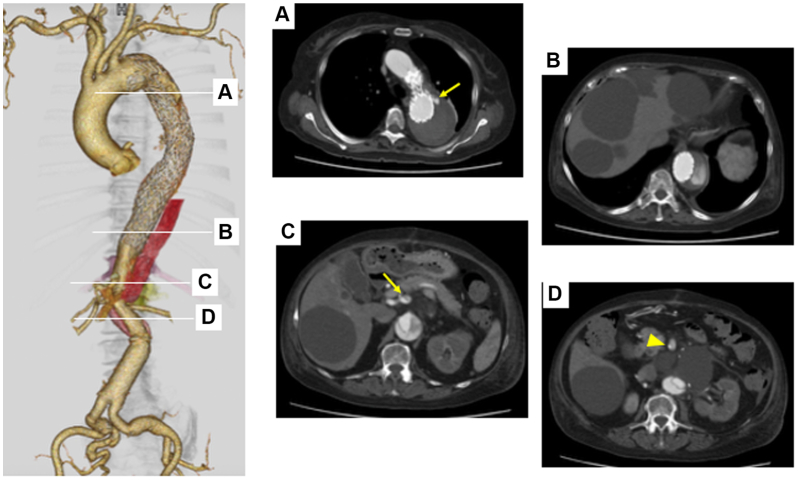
Contrast-enhanced computed tomography (CT) reveals progressive false lumen (FL) enlargement due to a type Ia endoleak after thoracic endovascular aortic repair (TEVAR) (A, *arrow*) and persistent retrograde flow (B) from multiple re-entries involving the celiacomesenteric trunk (C,*arrow*; D,*arrowhead*).
